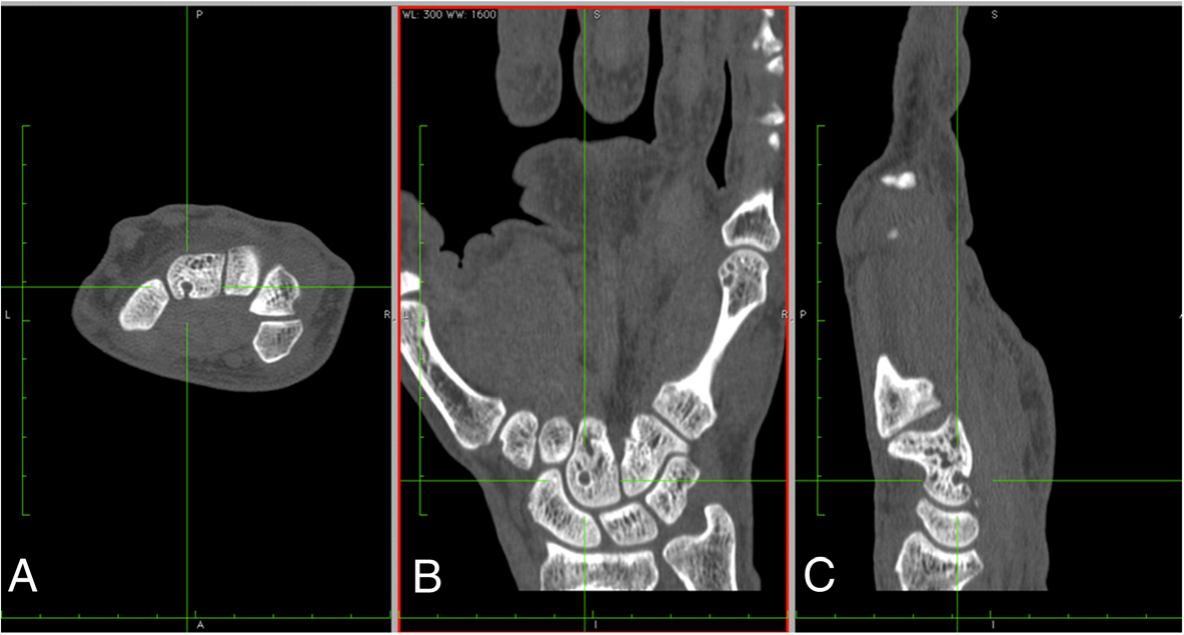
An example of bone erosion of the capitate bone seen on axial (A), coronal (B) and sagittal (C) CT reconstructions of wrist.
